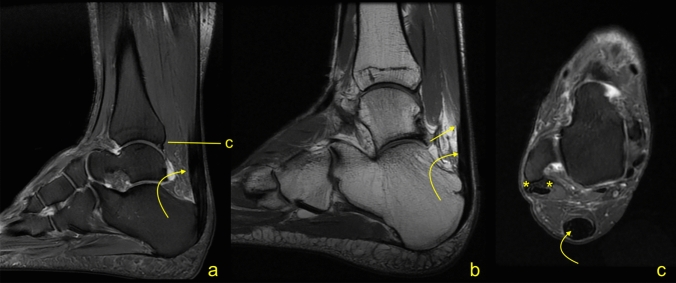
A 45-year-old male with painful Achilles tendinopathy and a short free tendon measuring 28.3 mm. MRI (a PD−w fat sat, b PD−w, c PD−w fat sat) revealed convex outline of the Achilles tendon (curved arrow). MRI revealed split rupture of the peroneus brevis (asterisk on c)
